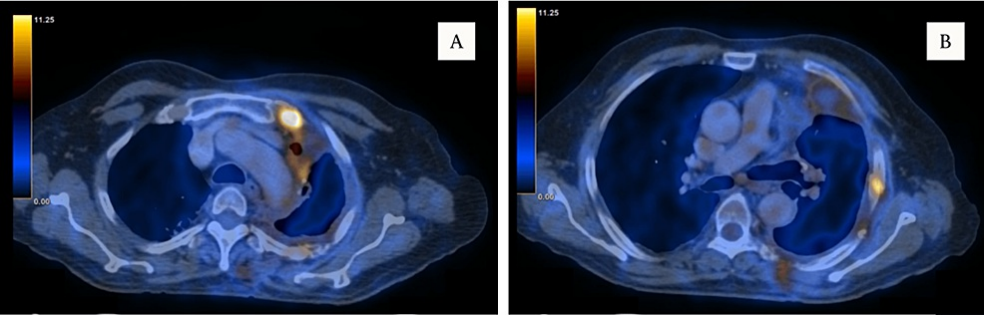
PET-CT scan (post-lobectomy) A) Axial section showing uptake in the surgical area (SUV max: 3.2) B) Axial section showing uptakes in subcarinal node and left ribs
